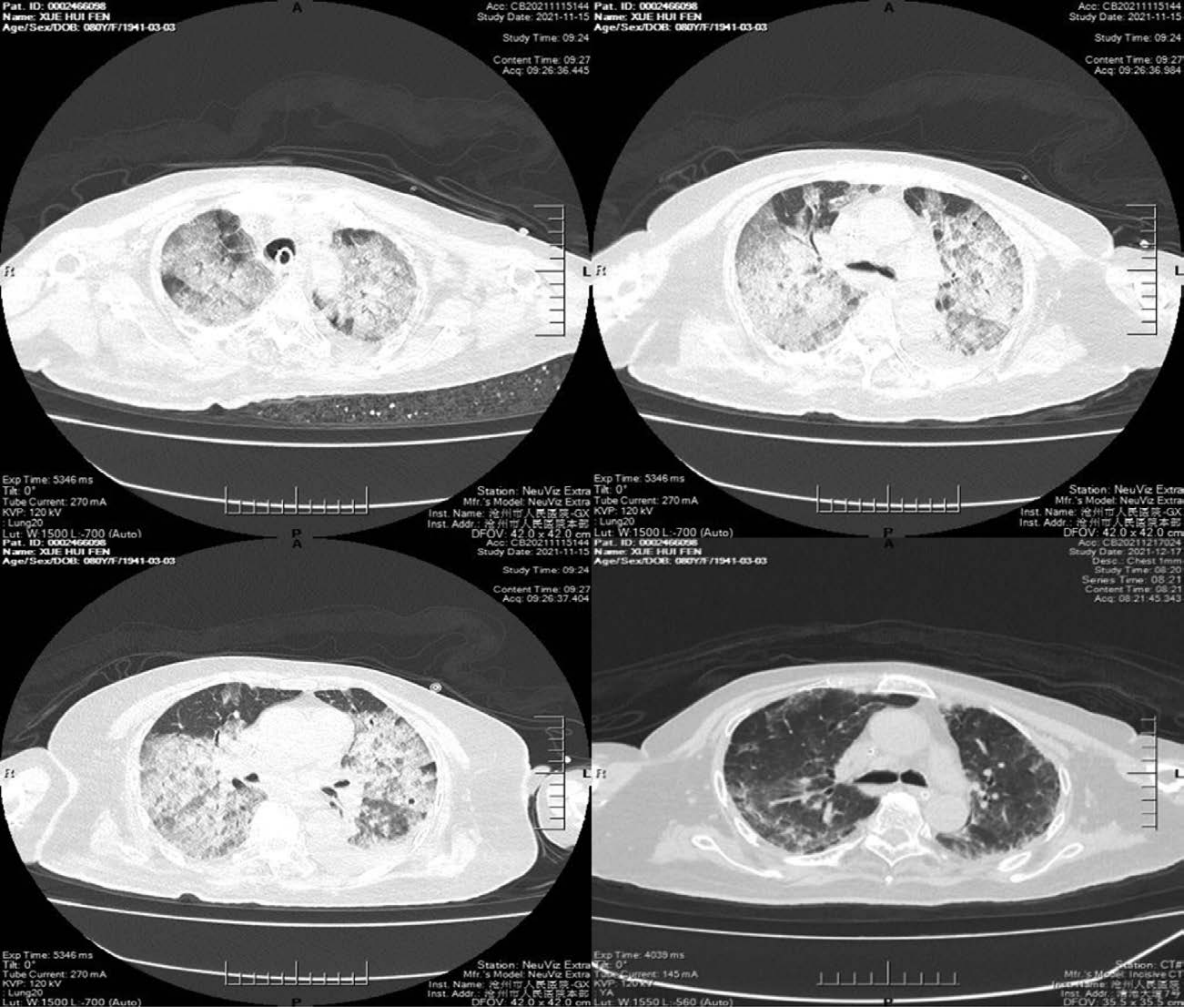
Results of CT scans of the lungs before anti-infective treatment showed the presence of bilateral pneumonia, enlarged pulmonary artery, and left pleural effusion. CT = computed tomography.
